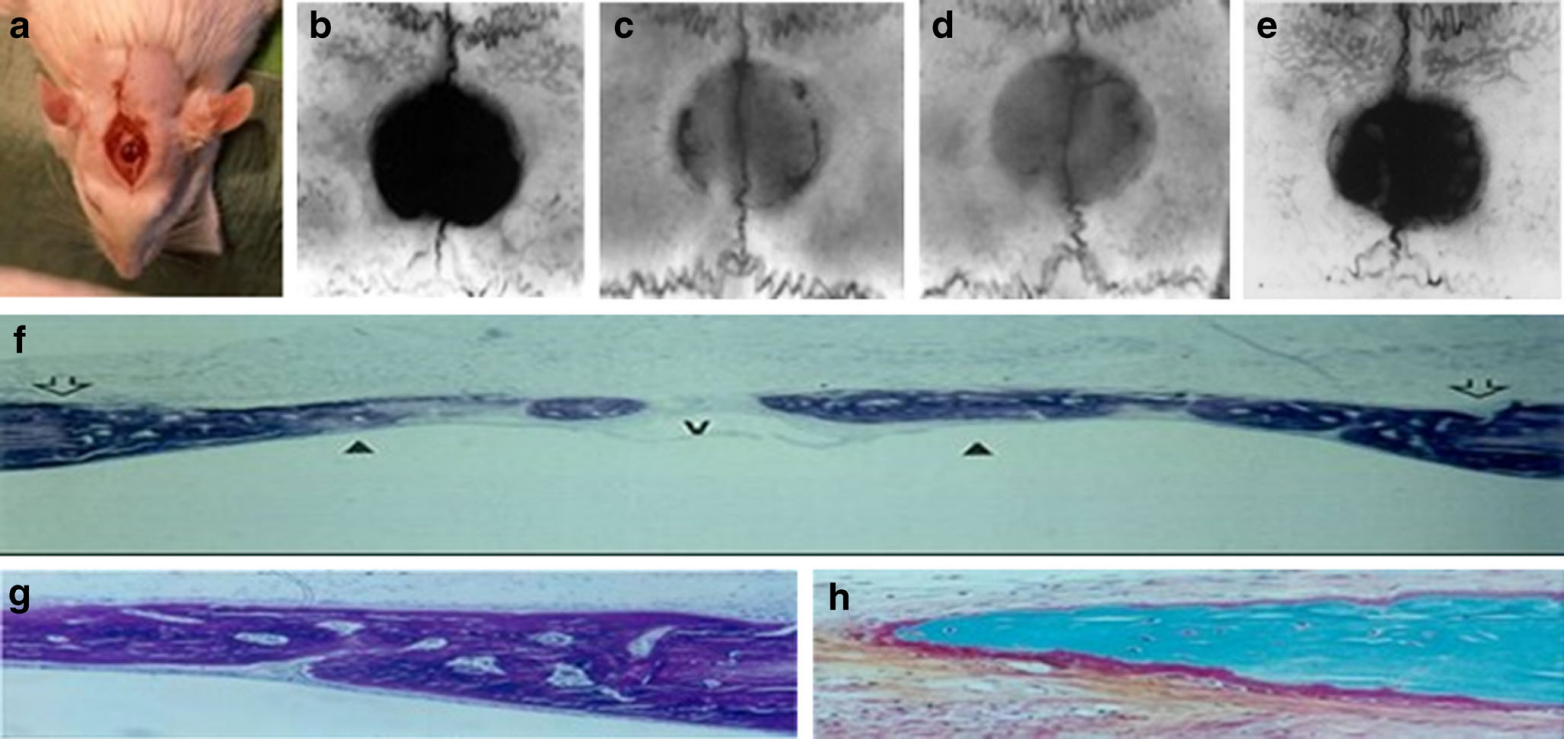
Filling of craniotomy defects. A. Operative view of the defect. X-ray of defects treated with a collagen plaster soaked in 30 ul of B. saline, C. RGTA^®^ solution (25 μg/ml) D. RGTA^®^ solution (100 μg/ml) and E. RGTA^®^ solution (400 ug/ml), after 4 weeks. F. Full macroscopic view of the skull defect with top arrows showing both sides of the defect and bottom arrows indicating new bone formation. Note the convex shape of the new bone structure, matching with expected original bone. v at the center indicates the saggital vein. G. Enlargement showing the lamellar organization of the flat bone as expected from a skull. H. alkaline phosphatase positive osteoblastic cells
